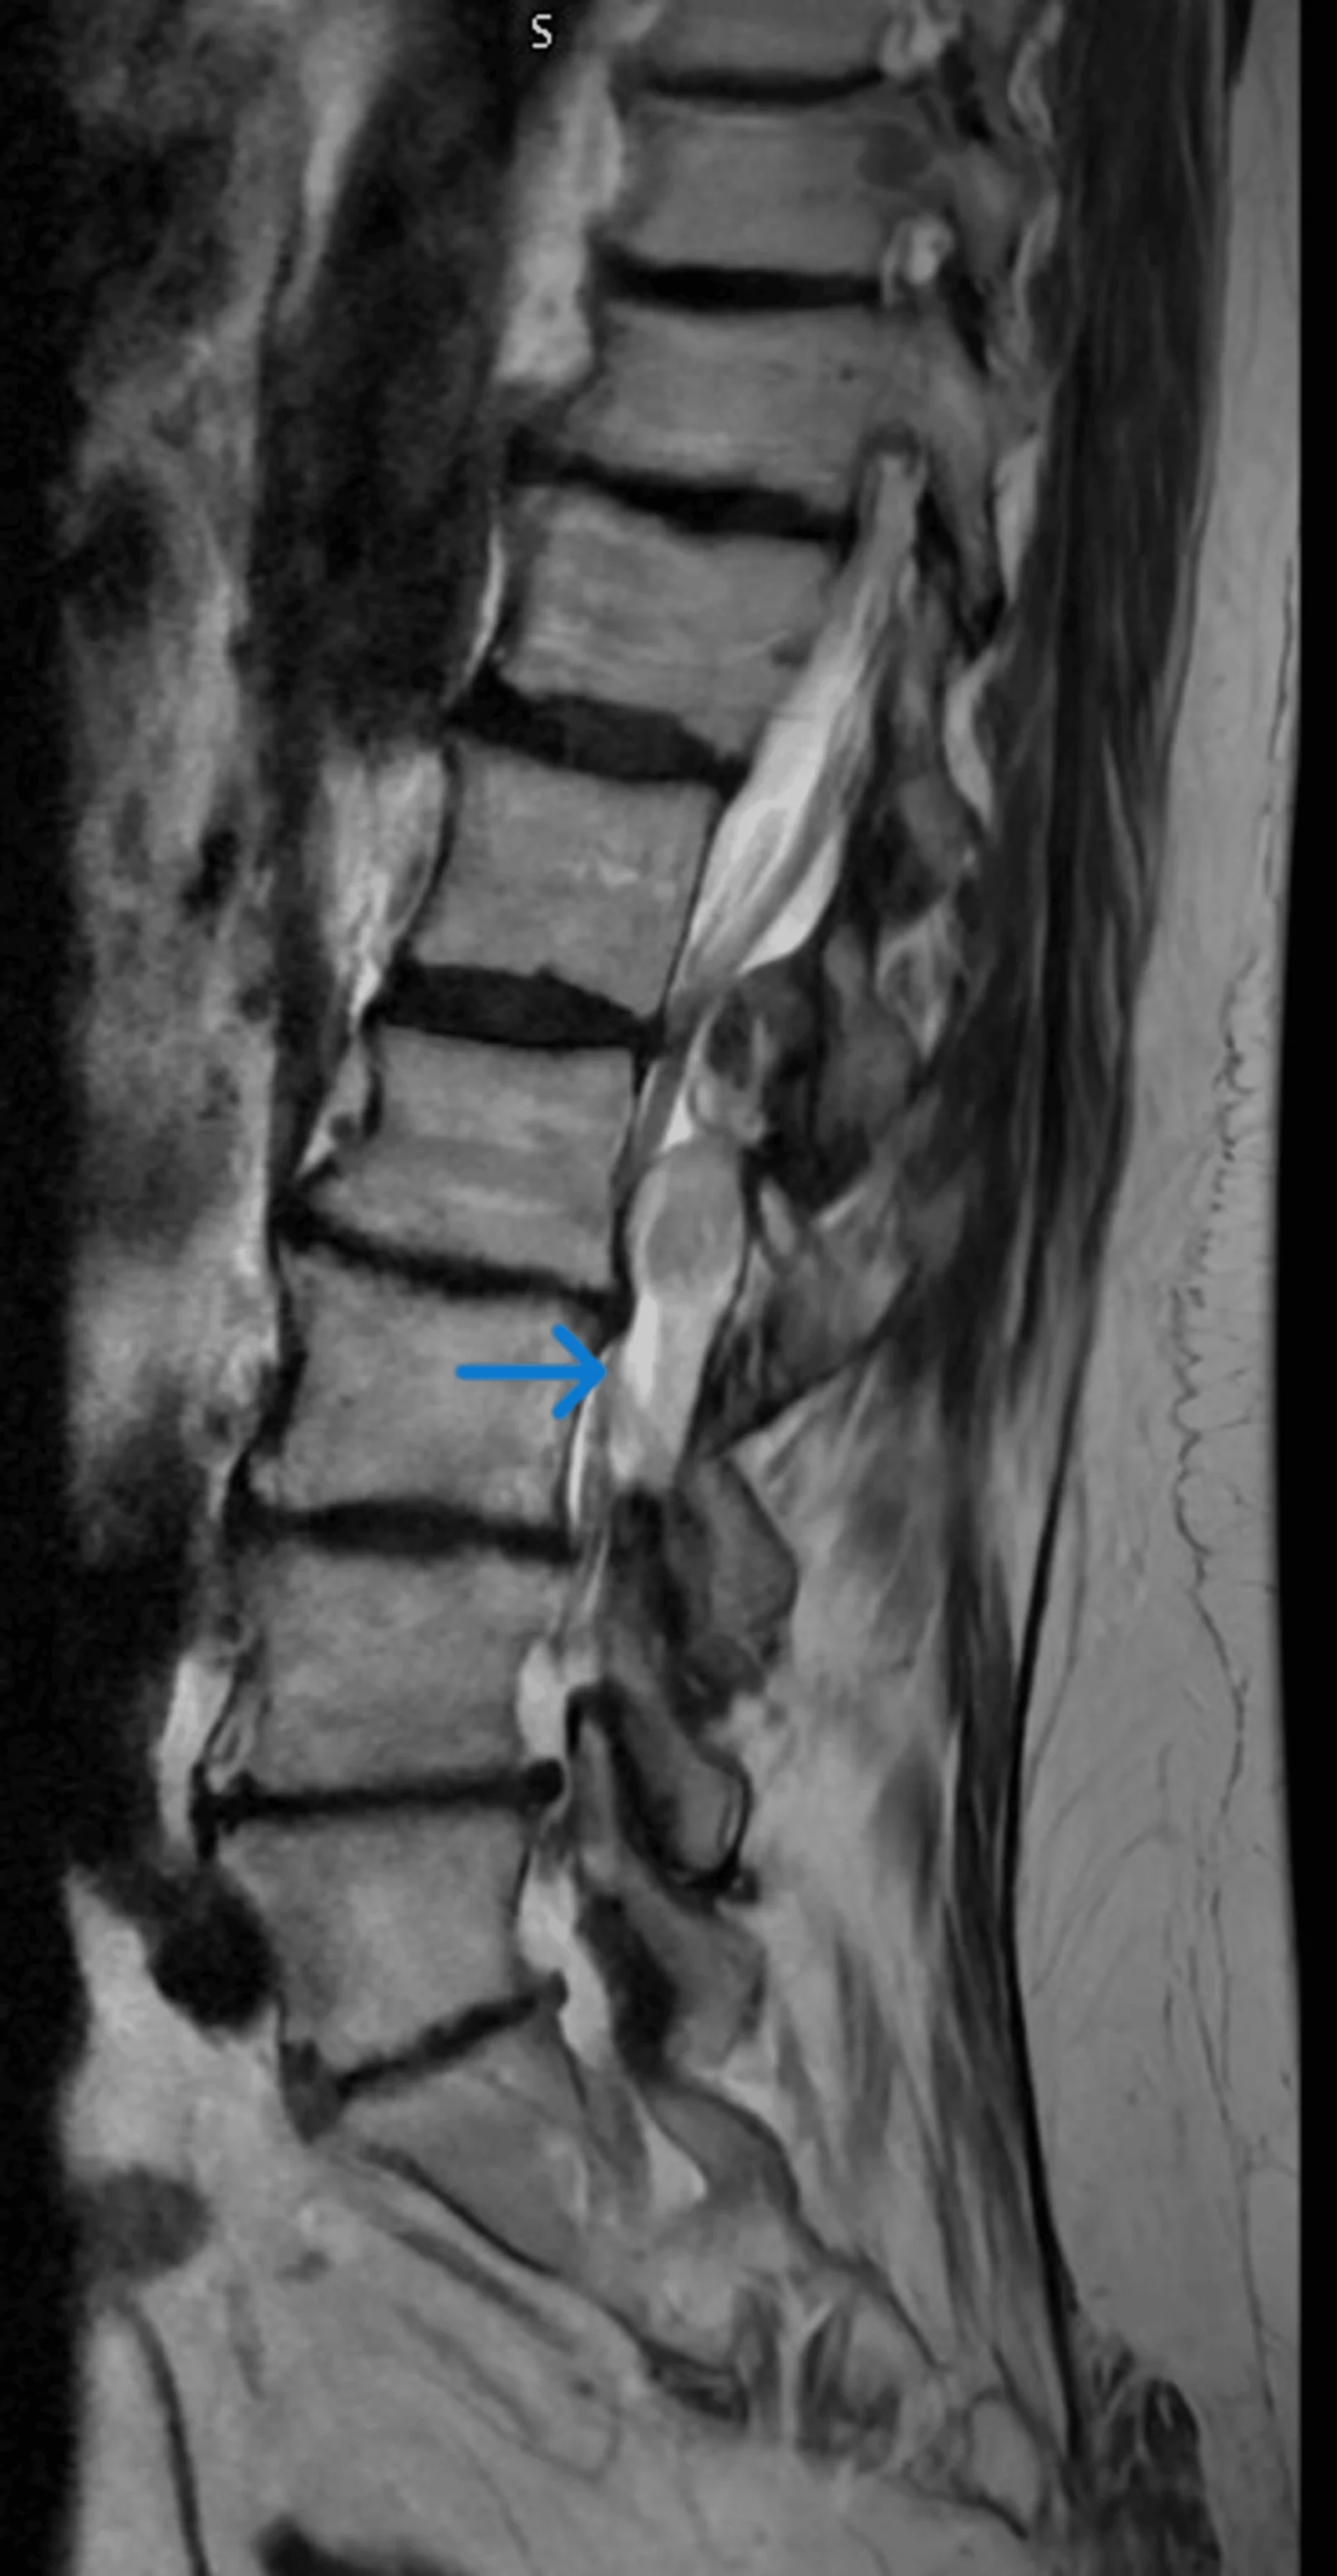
Sagittal T2-weighted MRI of the lumbar spine Sagittal T2‑weighted MRI demonstrating a dorsal hyperintense collection from L1 to L3 compressing the thecal sac (arrow).
